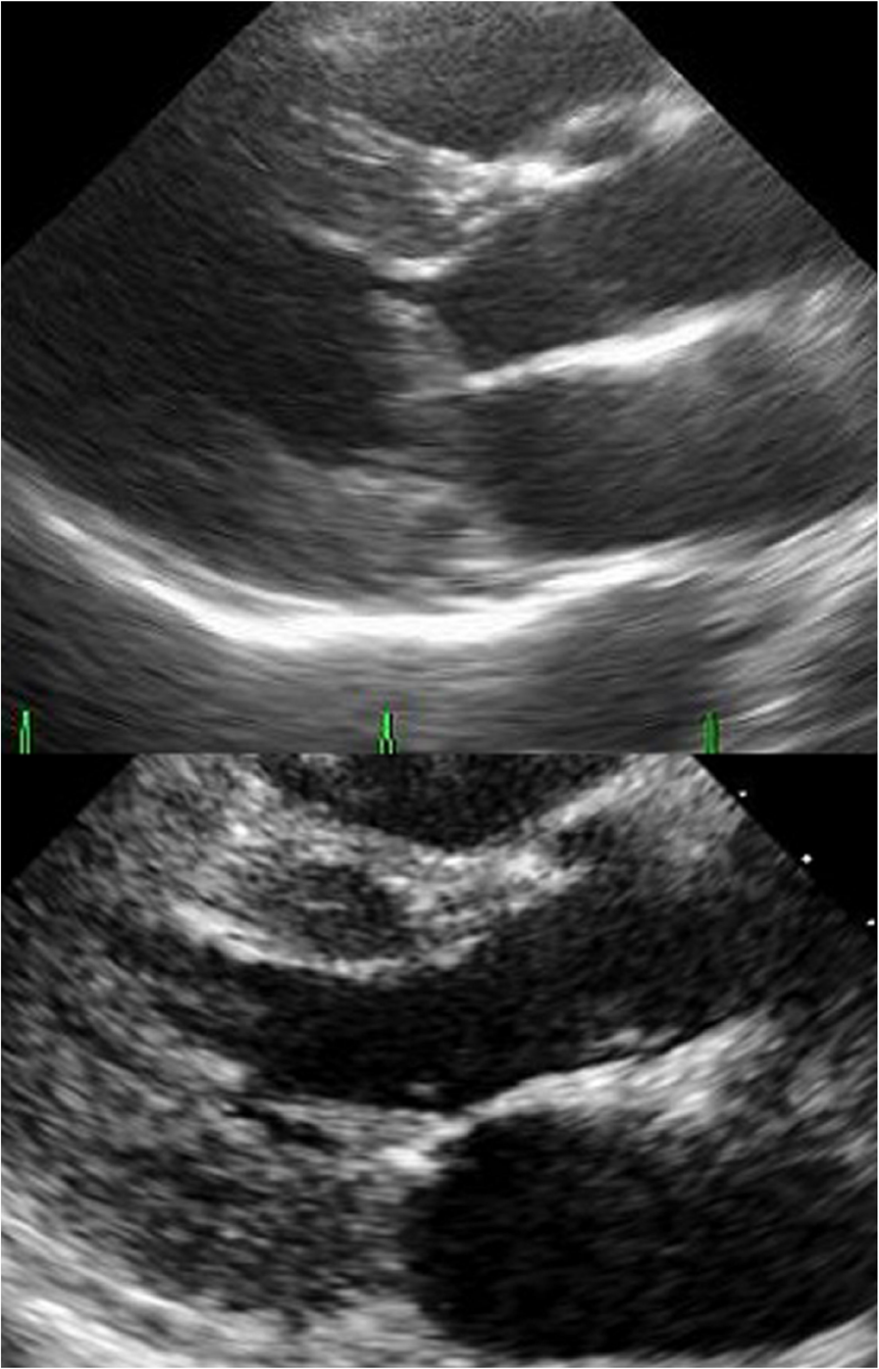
(Top) Preoperative transthoracic echocardiography revealed a left ventricular outflow obstruction caused by systolic anterior motion of the anterior mitral leaflet. The septal thickness was 17 mm at the contact point. (Lower) Postoperatively, echocardiography showed the absence of systolic anterior motion and mitral regurgitation. Accelerated flow in the left ventricular outflow tract was not detected
